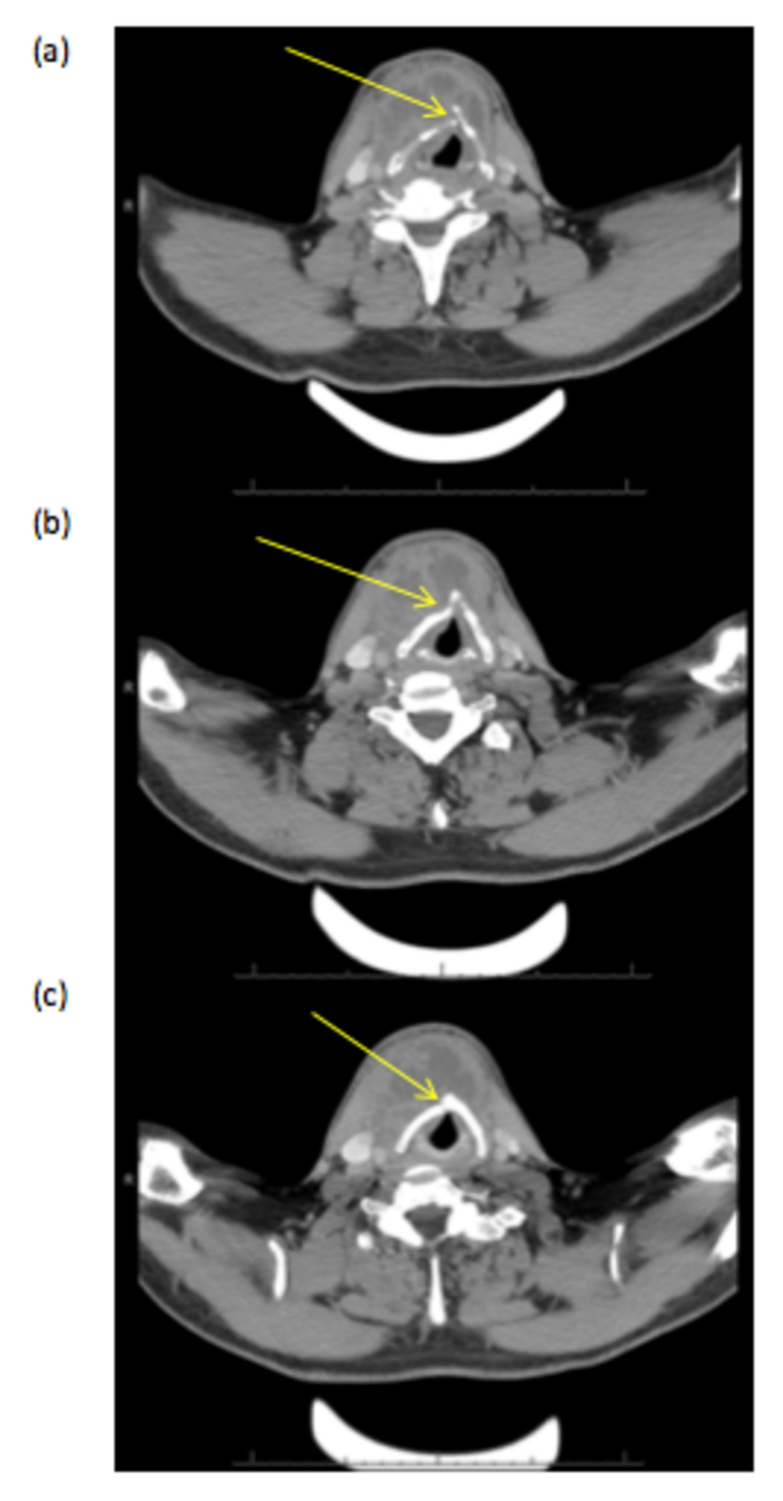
Axial sections (a-c) of contrast-enhanced CT scan demonstrating a vertical fracture of the thyroid cartilage (red arrows), with depression of the right thyroid lamina posteriorly due to anterior neck collection.
